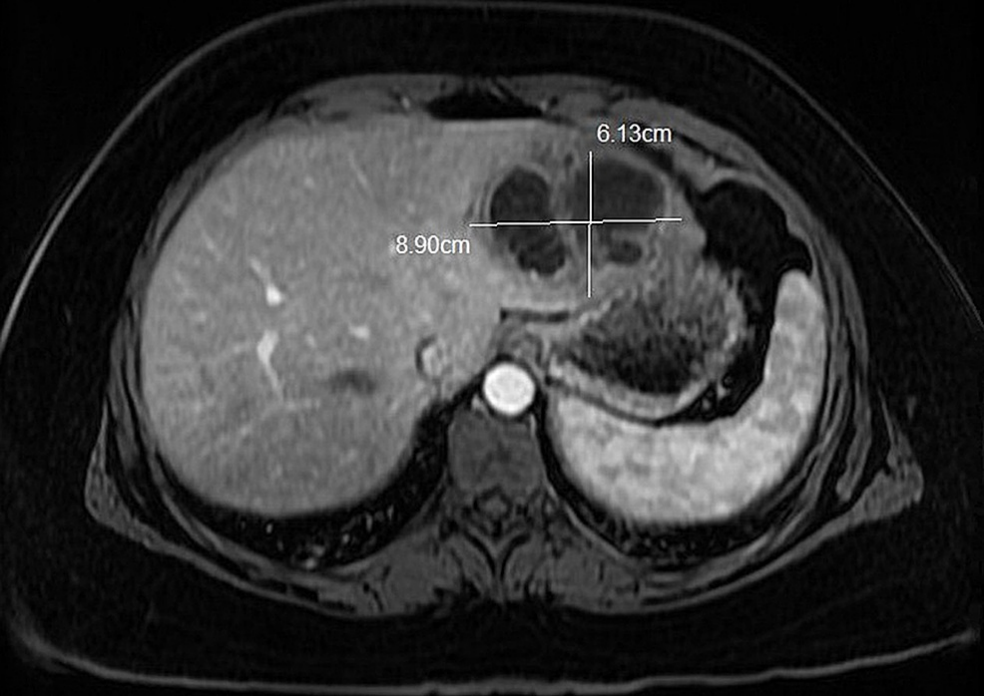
T-1 MRI abdomen with an annotated hepatic abscess in the lower left lobe
